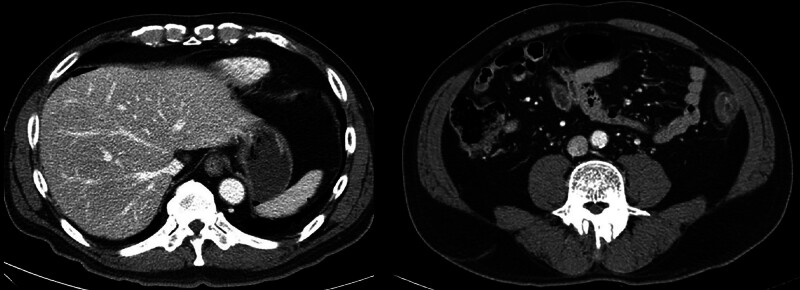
Abdominal computed tomography on day 54 showing free intraperitoneal air around the liver and extensive colonic necrosis, indicating delayed post-polypectomy perforation.
